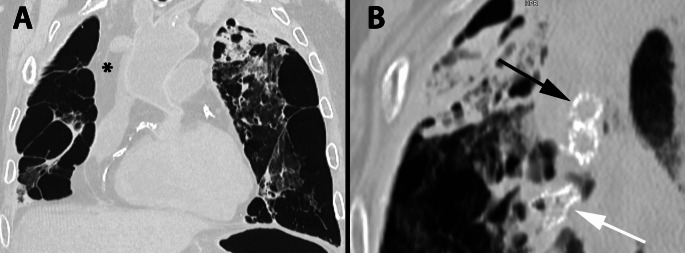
Post-mortem CT scan of the chest (lung kernal and windows; slice thickness 0.3 mm) showing severe emphysematous change and bullous transformation (most prominent on the right side post lobectomy); * marks right-sided fluid accumulation; also note calcification of the coronary arteries (A). Three endobronchial valves (present in the apical region of the left lung); Black arrow– valves in cross-section. White arrow– valve in longitudinal view (B)
